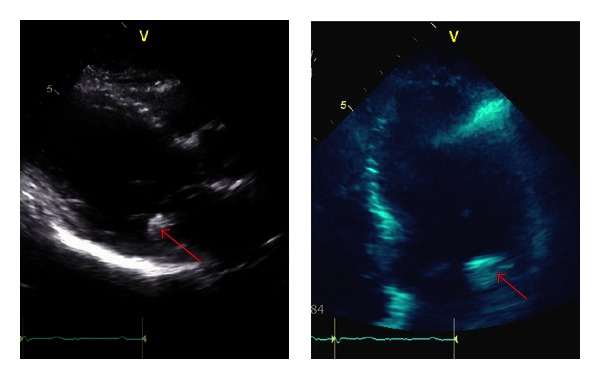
Transthoracic echo images demonstrating oval, heavily calcified echo-density on posterior mitral annulus that is difficult to differentiate from MAC (see arrow).
